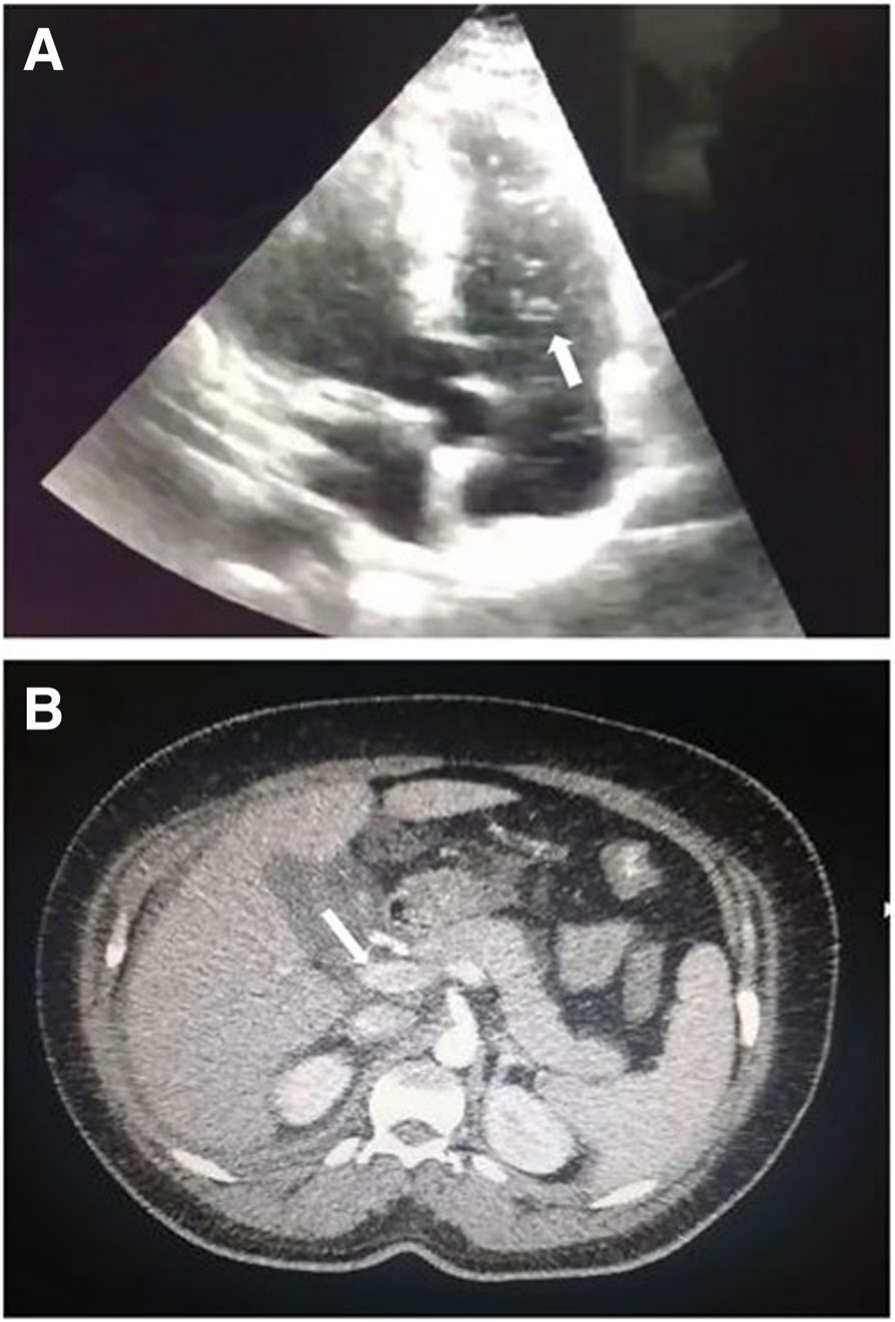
Diagnostic Images performed. A Transthoracic echocardiogram showing the presence of bubbles in the right chambers (white arrow). B Contrast-enhanced abdominal tomography showing acute non-cirrhotic portal vein thrombosis: partial enhancement of the portal vein, showing an image compatible with a thrombus inside (white arrow)
